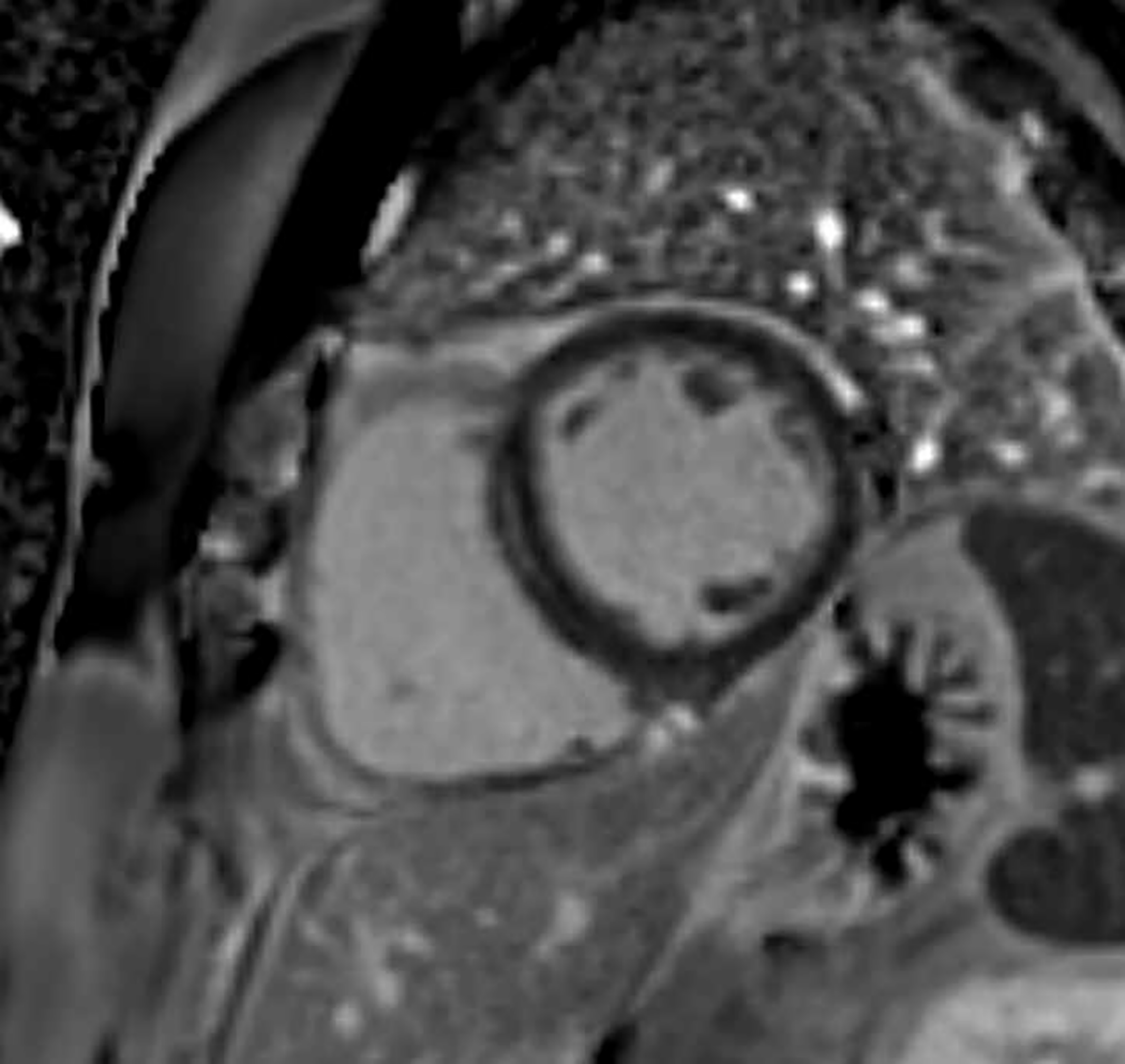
Atypical pattern of late gadolinium enhancement detected with cardiac magnetic resonance imaging in a 50-year-old male. Linear mid-myocardial wall enhancement is noted in mid to basal interventricular septum. His coronary artery calcium score was 0.
